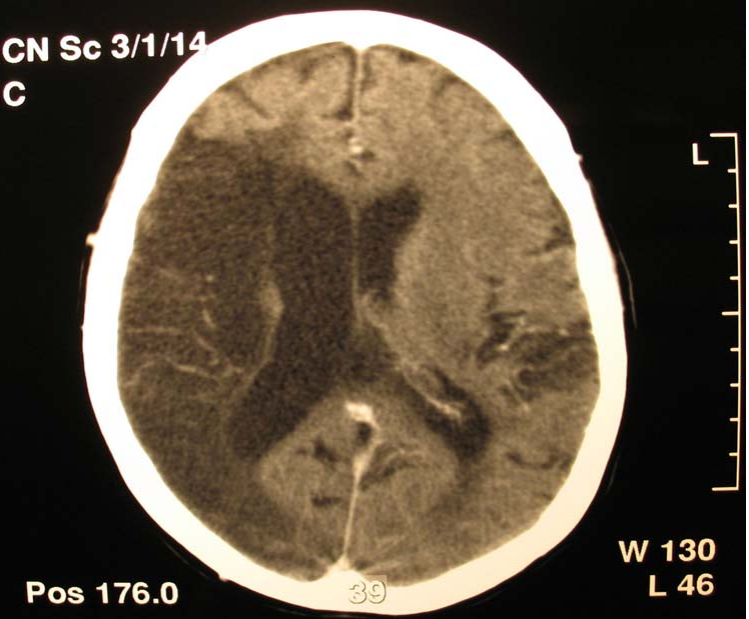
Preoperative CT brain scan Sample figure title. CT brain scan-Old ischemic findings in the right hemisphere.
